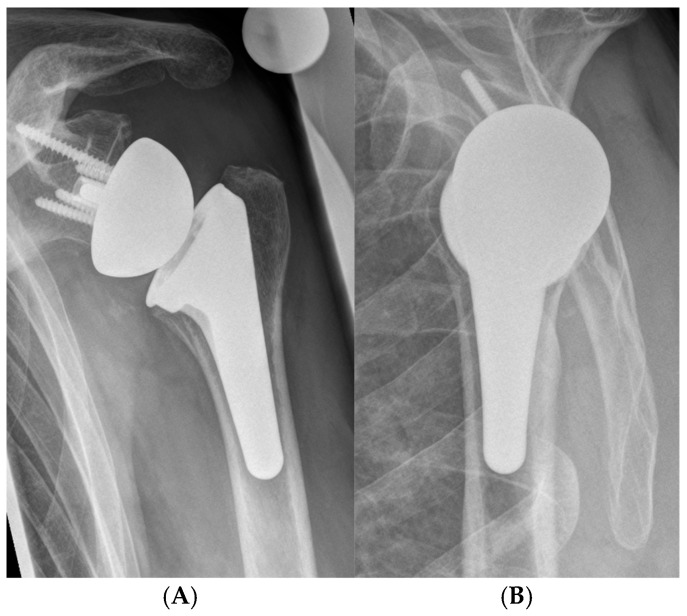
Postoperative radiograph showing a cementless short stem reverse shoulder arthroplasty in a.p. (A) and y-view (B).
